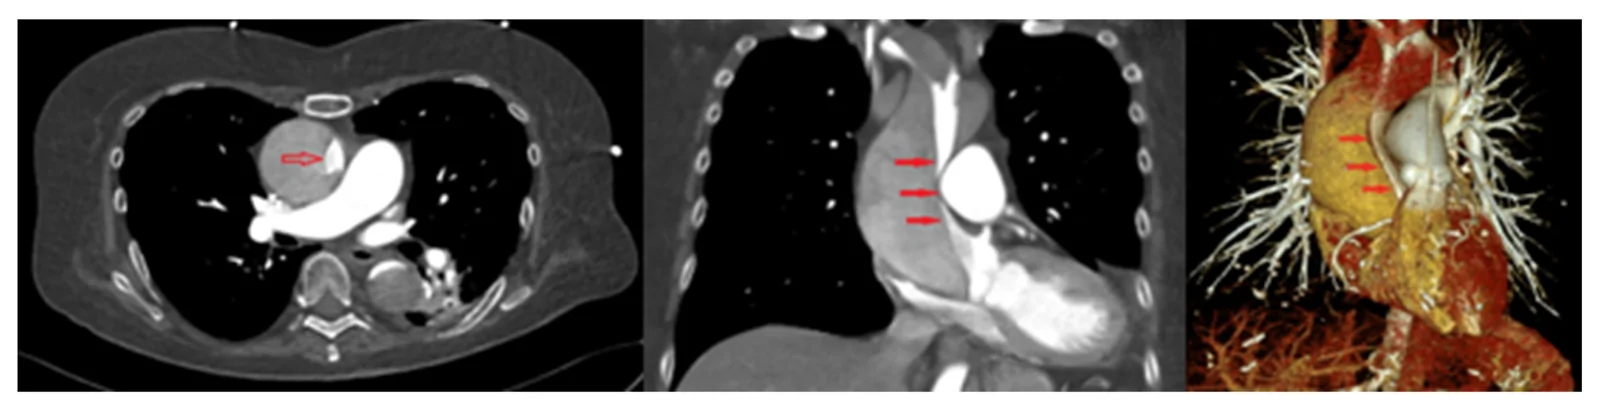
CT imaging after six weeks demonstrating the remaining true lumen of the aorta.
